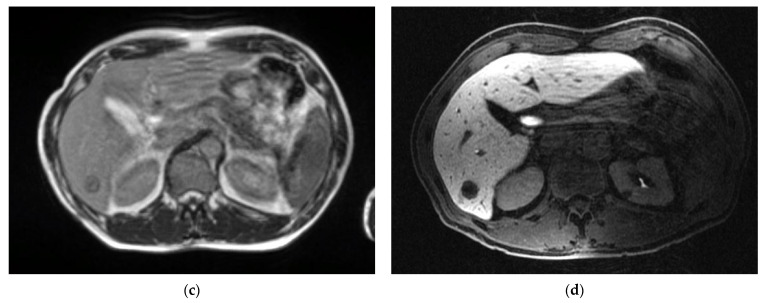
Comparison of different imaging modalities for the soft tissues of the abdomen. (a) Axial CBCT imaging of the liver taken on a conventional linear accelerator. (b) Axial CT slice of the abdomen used for planning with each organ of a similar electron density. (c) Axial slice of the abdomen acquired with 0.35 T MRI. (d) Axial slice of the abdomen using 1.5 T MRI. Lesion identification is much easier on both 0.35 and 1.5 T MRI images compared to CT and CBCT scans.
